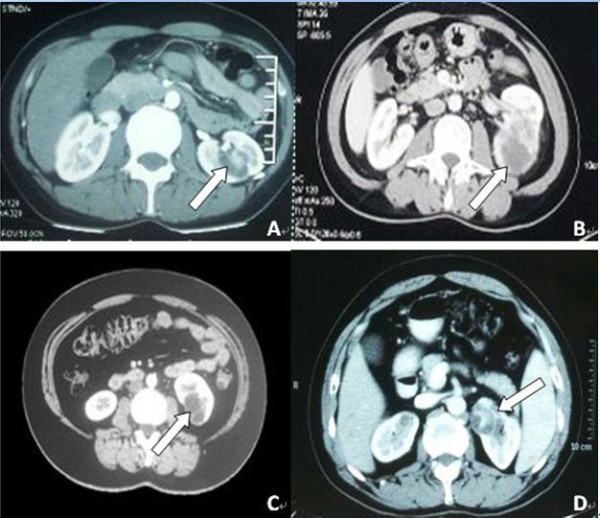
Preoperative CT scans of the abdomen revealed a typical MCRCC (white arrow). (A) Typical images of MCRCC localized to the central location of the left kidney. (B) Typical images of MCRCC localized to the lower pole of the left kidney. (C) Typical images of MCRCC localized to the lower pole of the left kidney. (D) Typical images of MCRCC localized to the upper pole of the left kidney. CT, computed tomography; MCRCC, multilocular cystic renal cell carcinoma.
